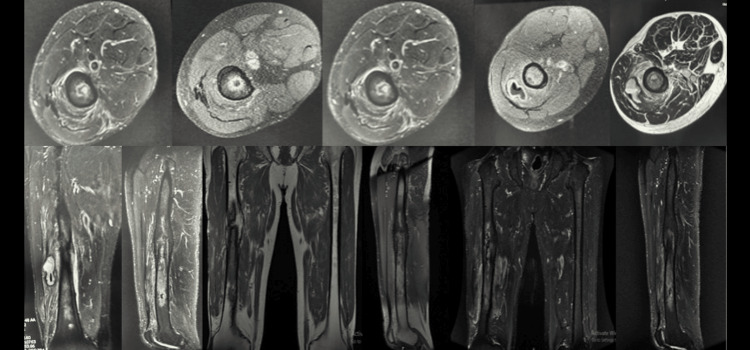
MRI of the thighs with axial, coronal, and sagittal views shows a 28×10 mm intraosseous formation with peripheral contrast enhancement consistent with an abscess, also seen in STIR views. MRI, magnetic resonance imaging
